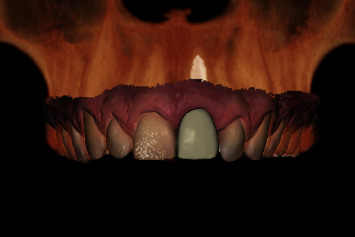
Digital set-up of the upper left central incisor, based on a superimposed intraoral scan and CBCT scan.
